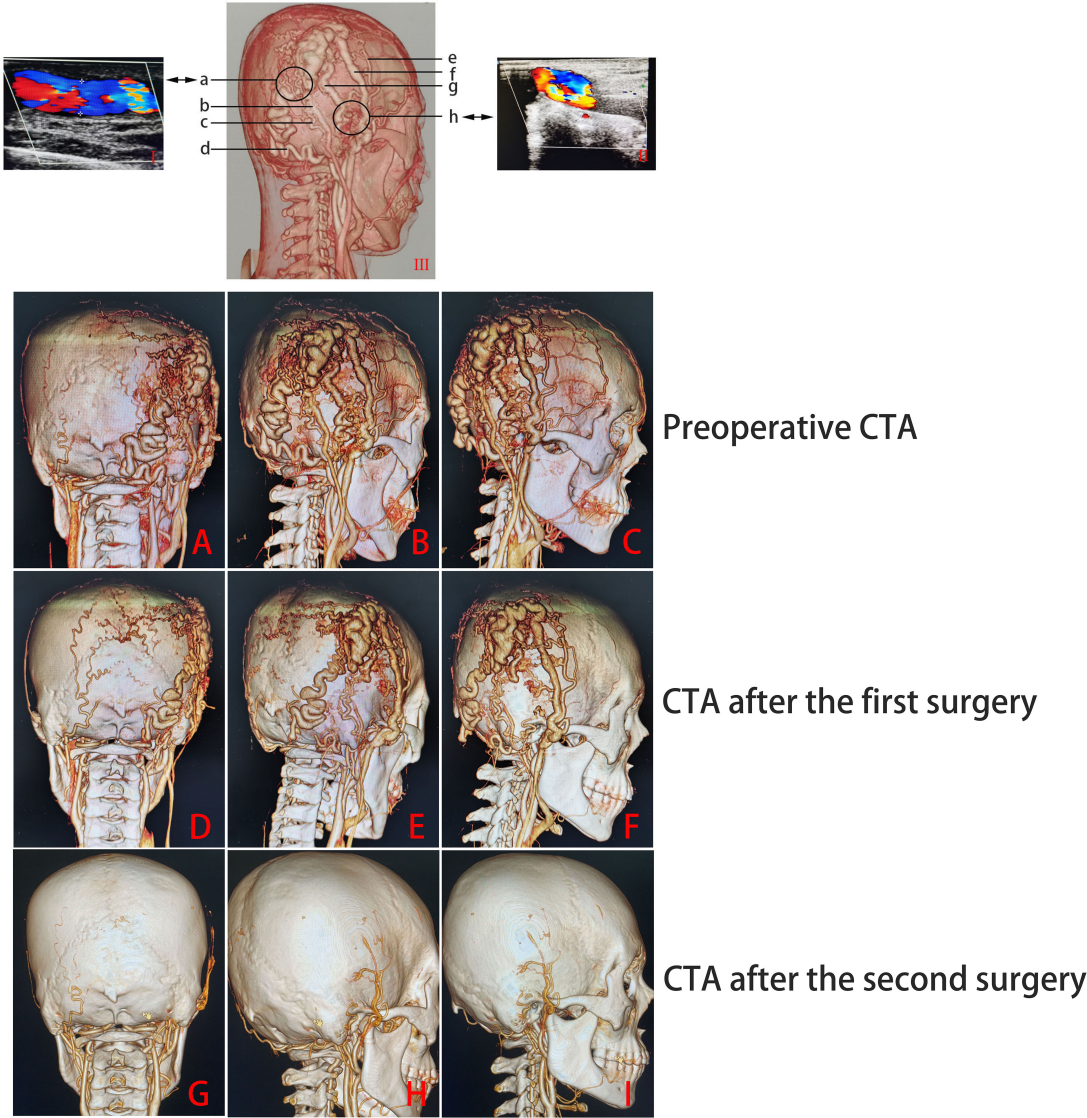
CTA and color Doppler ultrasound examination results (I/II, the results of color Doppler ultrasound; III, the results of the CTA examination; a: the fistula is related to the occipital artery; b, posterior auricular vein; c, posterior auricular artery; d, occipital artery; e, superficial temporal artery; f, superficial temporal vein; g, supplying artery; h, the fistula is related to the superficial temporal artery). Preoperative (A–C) and postoperative [first surgery (D–F); second surgery (G–I)] CTA examination results.
